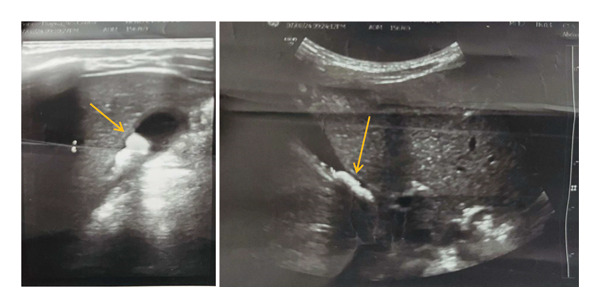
Sagittal and subcostal ultrasound views showing multiple gallstones within the gallbladder.
